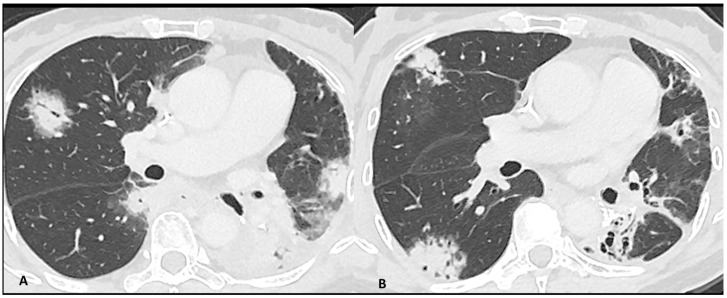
Immuno-related pneumonitis with typical organizing pneumonia features in a patient developed after four cycles of antiPD-1 treatment for lung cancer. The images at the pulmonary trunk depict (A) multiple bilateral peribronchovascular consolidations lobe as well as an extensive lower lobe consolidation. A 4-week CT follow-up shows the regression of the previous consolidation but the appearance of new ones (B), representing the “migratory” aspect of the organizing pneumonia.
